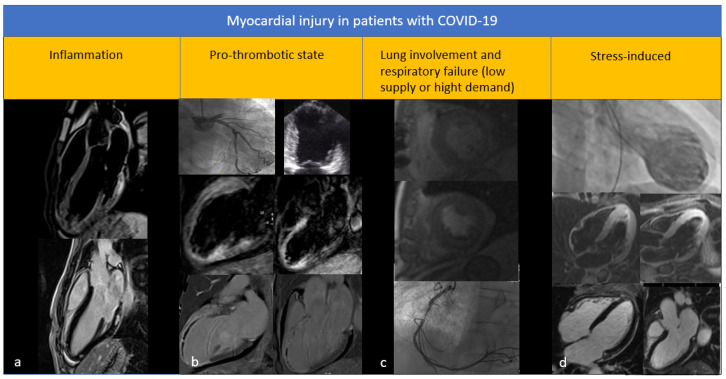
Myocardial injury in patients with COVID-19. (a) Case of myocarditis: CMR shows edema and fibrosis in the basal and apical infero-lateral wall of the left ventricle; (b) Case of myocardial infarction: coronary angiography shows occlusion of the anterior descending coronary artery; on echocardiography, alterations of the kinetics in the territory of the anterior descending coronary artery are noted and on CMR extensive areas of edema and fibrosis are described in the same location, with outcomes of microvascular damage; (c) Case of myocardial ischemia induced by hypoxia: stress CMR post-admission shows ischemia in the right coronary artery region, which will present a critical stenosis on coronary angiography; (d) Case of Tako-tsubo: ventriculography shows the typical apical ballooning appearance of the left ventricle; CMR confirms the presence of edema and the absence of fibrosis in the apical area.
